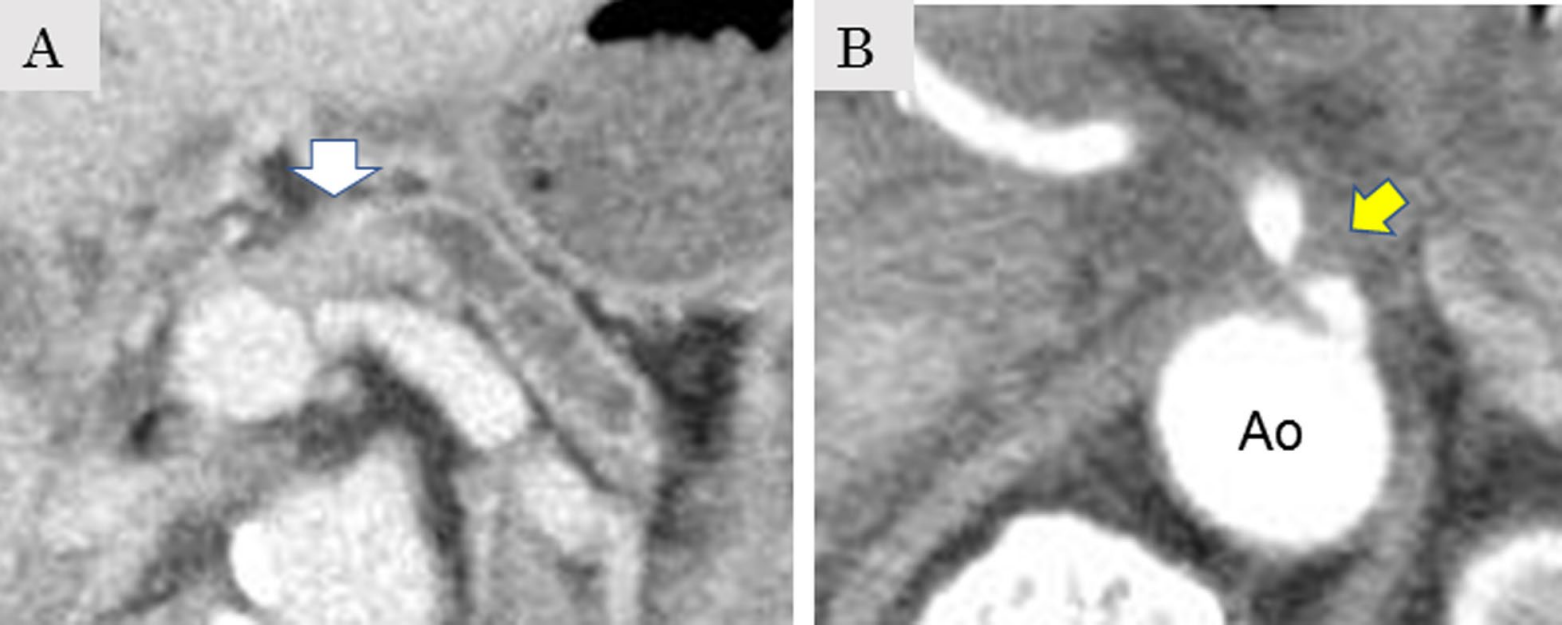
A Preoperative CT image which represents a resectable pancreatic cancer (white arrow). B Coincidental median arcuate ligament syndrome is shown (yellow arrow)
